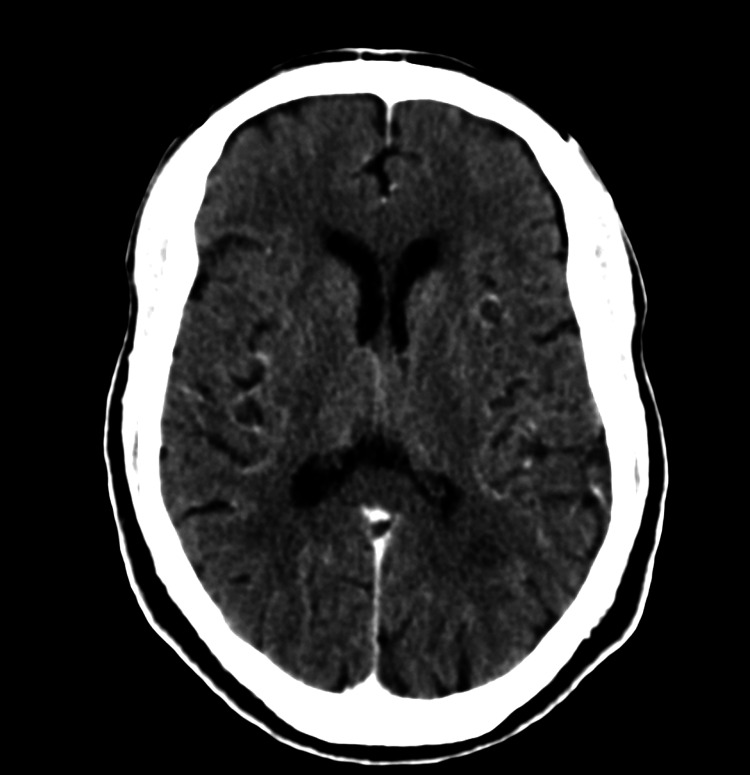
Non-contrast CT scan of the head one hour after coronary angiography
